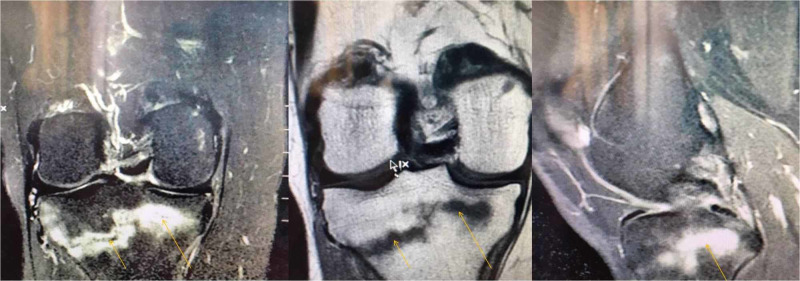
MRI of the right knee. The yellow arrows point to insufficiency fracture lines. Edema is obvious around the fracture lines.
